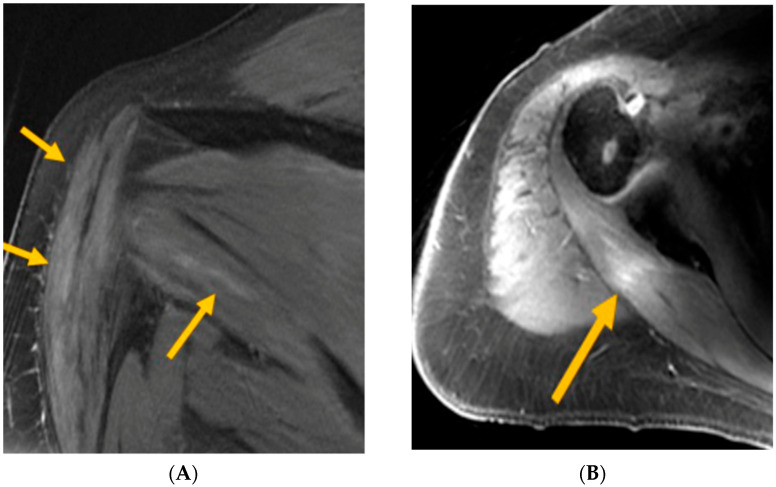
(A) MRI T2FS—arrows indicate deltoid and teres minor intramuscular edema (consistent with axillary neuropathy); (B) MRI T1FS—arrow indicates post-infraspinatus enhancement (consistent with upper trunk brachial plexopathy).
